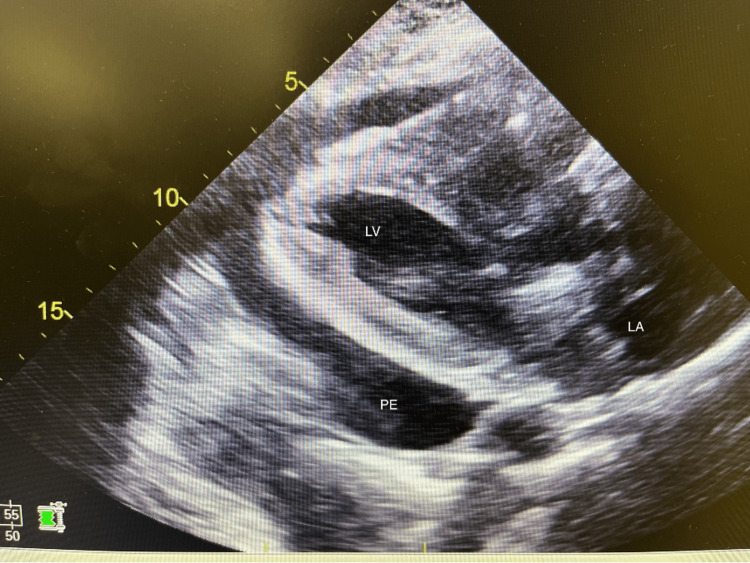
Long-axis view of the heart by 2D-echocardiogram with the confirmed presence of moderate to large pericardial effusion (PE), with clear compression of the left ventricle (LV) and left atrium (LA) collapse
